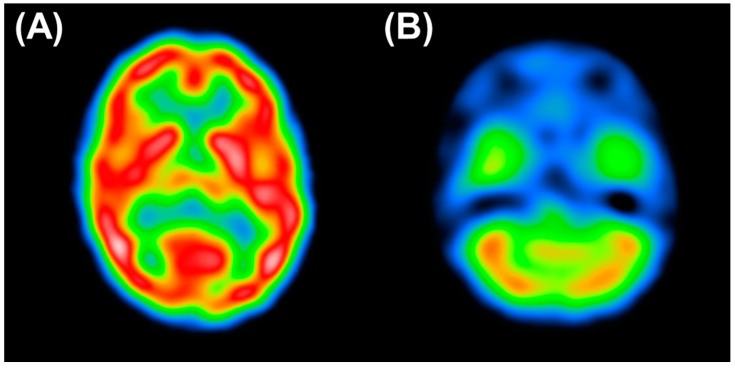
Cerebral blood flow (CBF) evaluated by ^99m^Tc-ethyl cysteinate dimer single photon emission computed tomography (SPECT). (A) No significant CBF abnormality in the cerebrum and basal ganglia is seen. (B) SPECT shows mild decrease in CBF in the cerebellum.
